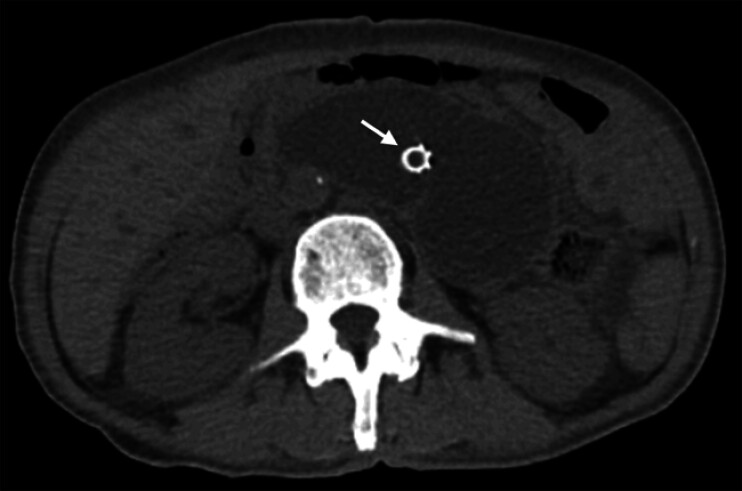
Preprocedural computed tomography (CT) scan imaging. Preprocedural CT revealed the dilation of the afferent loop in a 61-year-old man who had undergone total gastrectomy and esophagojejunostomy (Roux-en-Y) for gastric signet ring cell carcinoma three years previously. The patient had a biliary stent for obstructive jaundice (white arrow).
